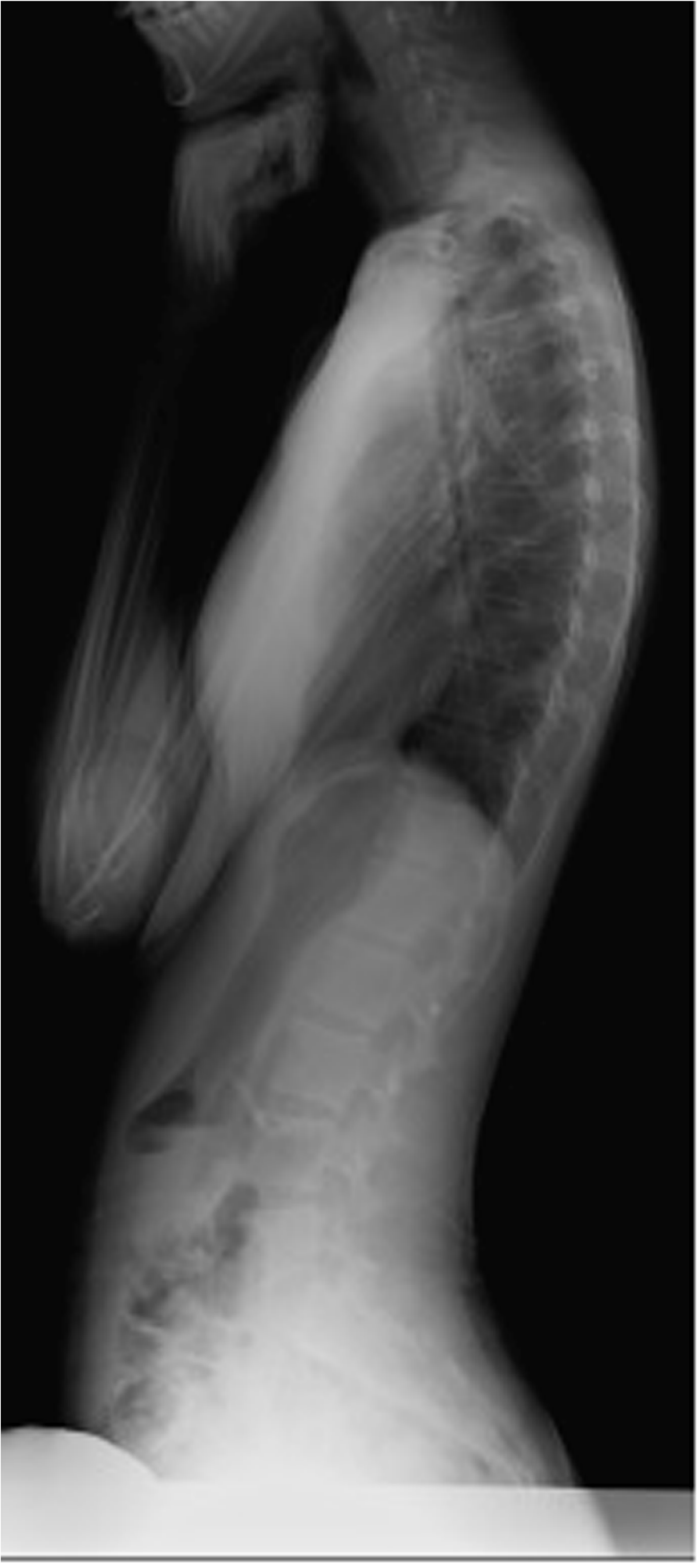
Representative lateral X-rays demonstrate that the evaluation of the femoral head is not possible in the lateral view
